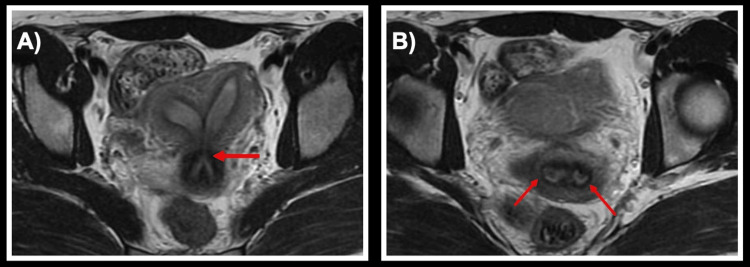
MRI demonstrating uterine didelphys and cervix bicollis (A) Two distinct uterine cavities with an area of communication (indicated by an arrow). (B) Two distinct cervical canals (each canal indicated by an arrow).
